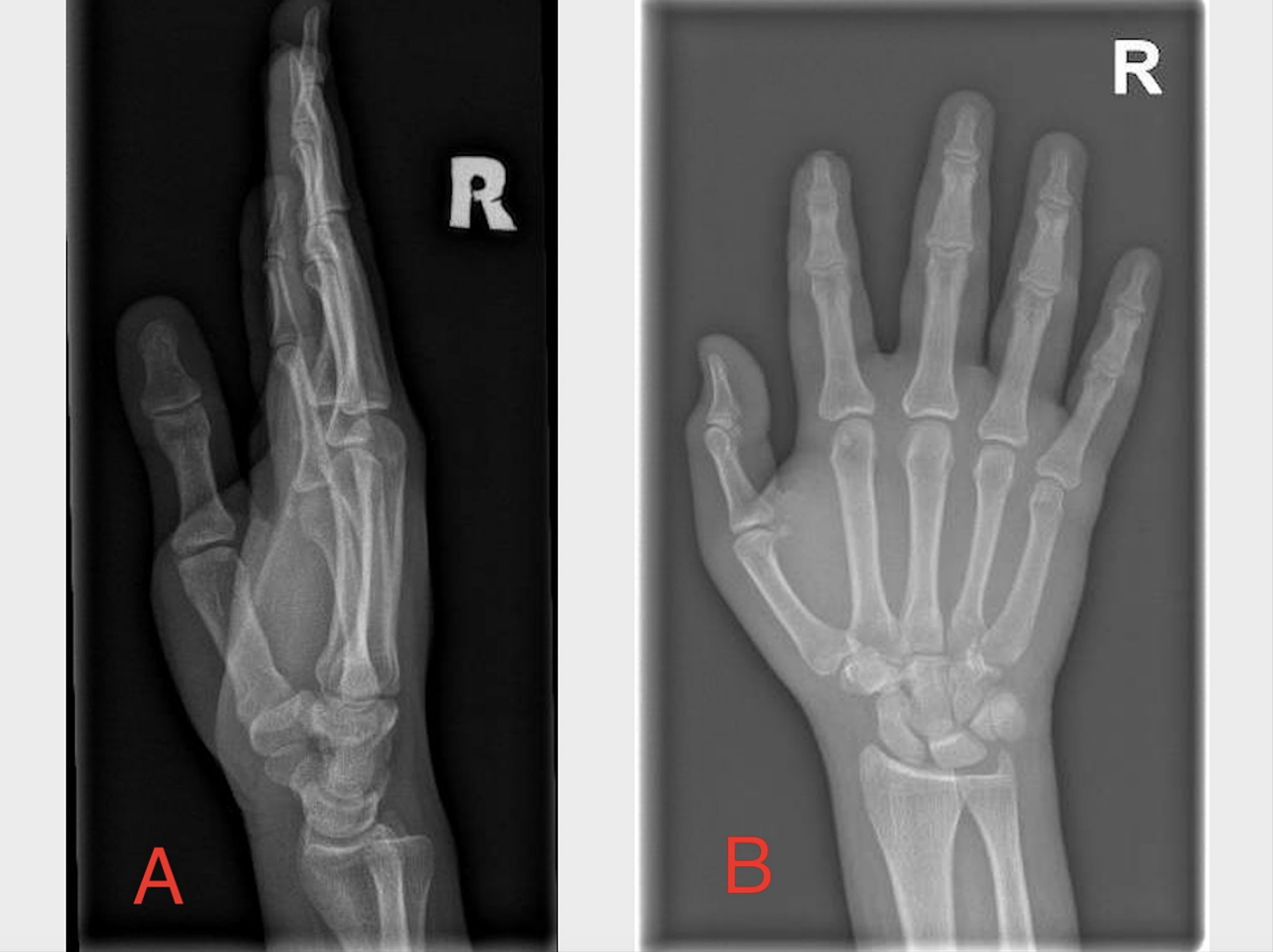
Right hand X-ray showing soft tissue swelling, no foreign body noted. A: lateral view, B: anteroposterior view
